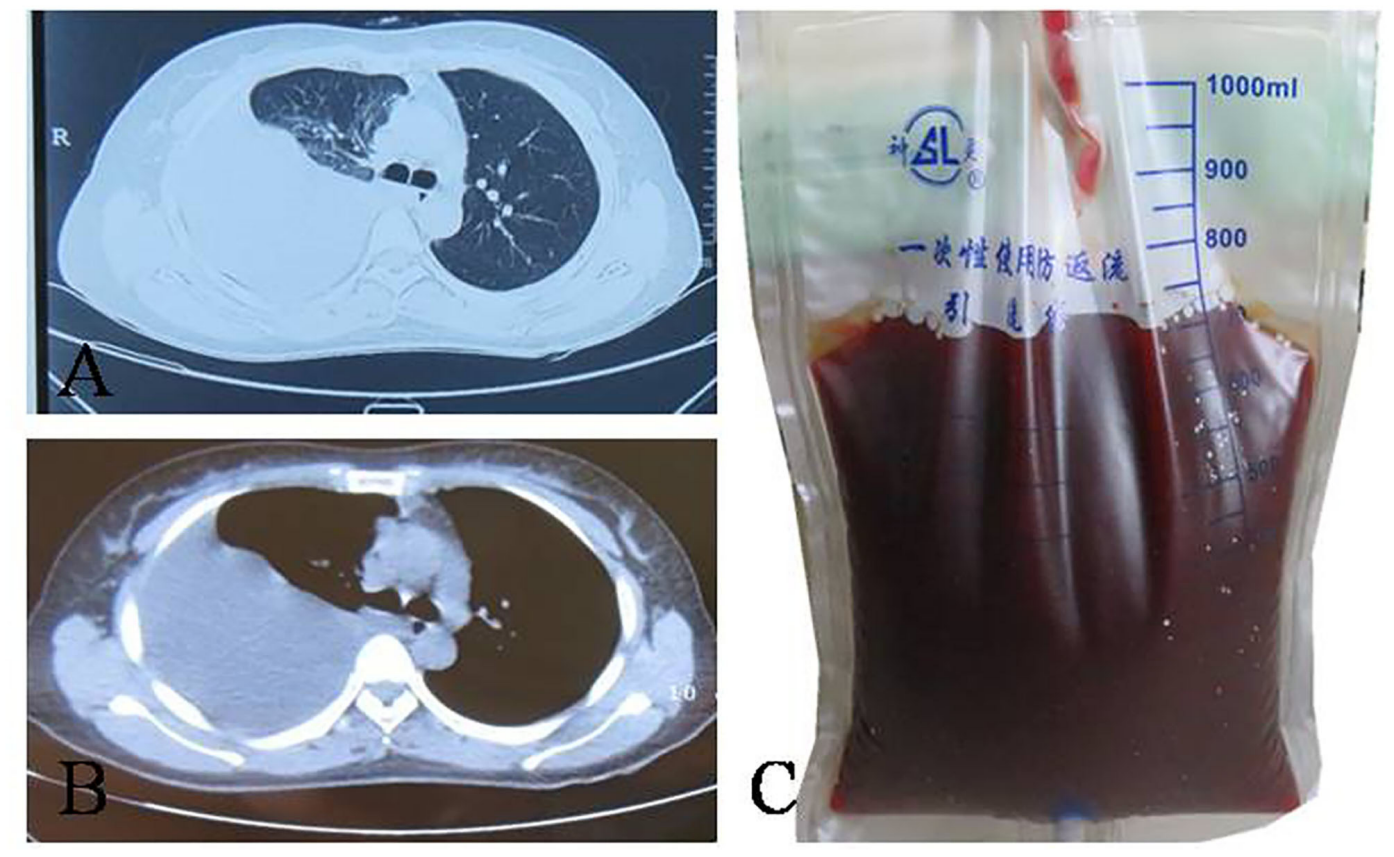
Computed tomography manifestation and pleural fluid of the patient. (A,B) right-sided pleural effusion; (C) haemorragic fluid aspirated from right pleural cavity.
